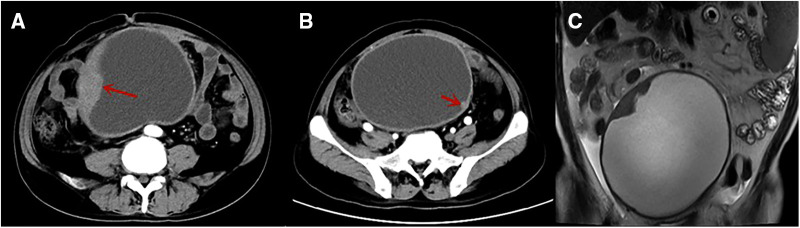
Ct scan showing a large cystic tumor in the lower abdomen with an unevenly thickened cystic wall, the solid component of the tumor (red arrow, A), and mural nodules (red arrow, B) with enhancement in the arterial phase. MRI revealed a large cystic tumor with an internal fluid signal (C). CT, computed tomography; MRI, magnetic resonance imaging.
